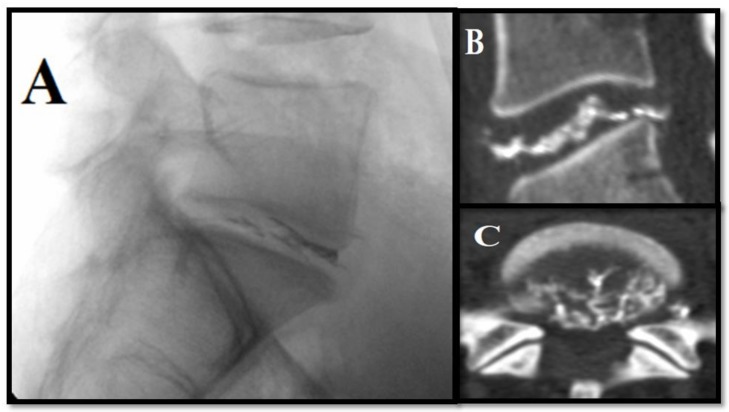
Radiopaque gelified ethanol for L5–S1 disc herniation: (A) Lateral fluoroscopic view: notice radiopaque gel in the L5–S1 disc. (B,C) Post-intervention follow-up CT exam shows optimal intradiscal distribution of the solution.
